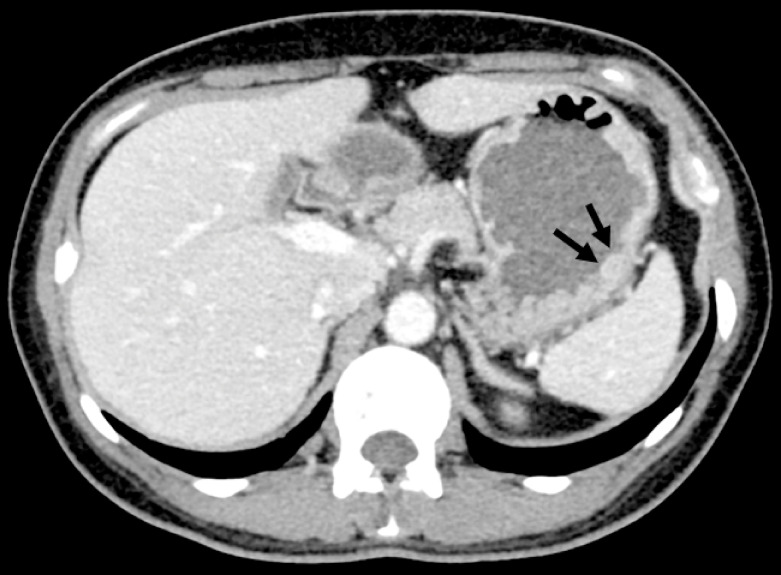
Enhanced computed tomography confirmed the lesion with an endoluminal growth pattern in the gastric body (arrows).
